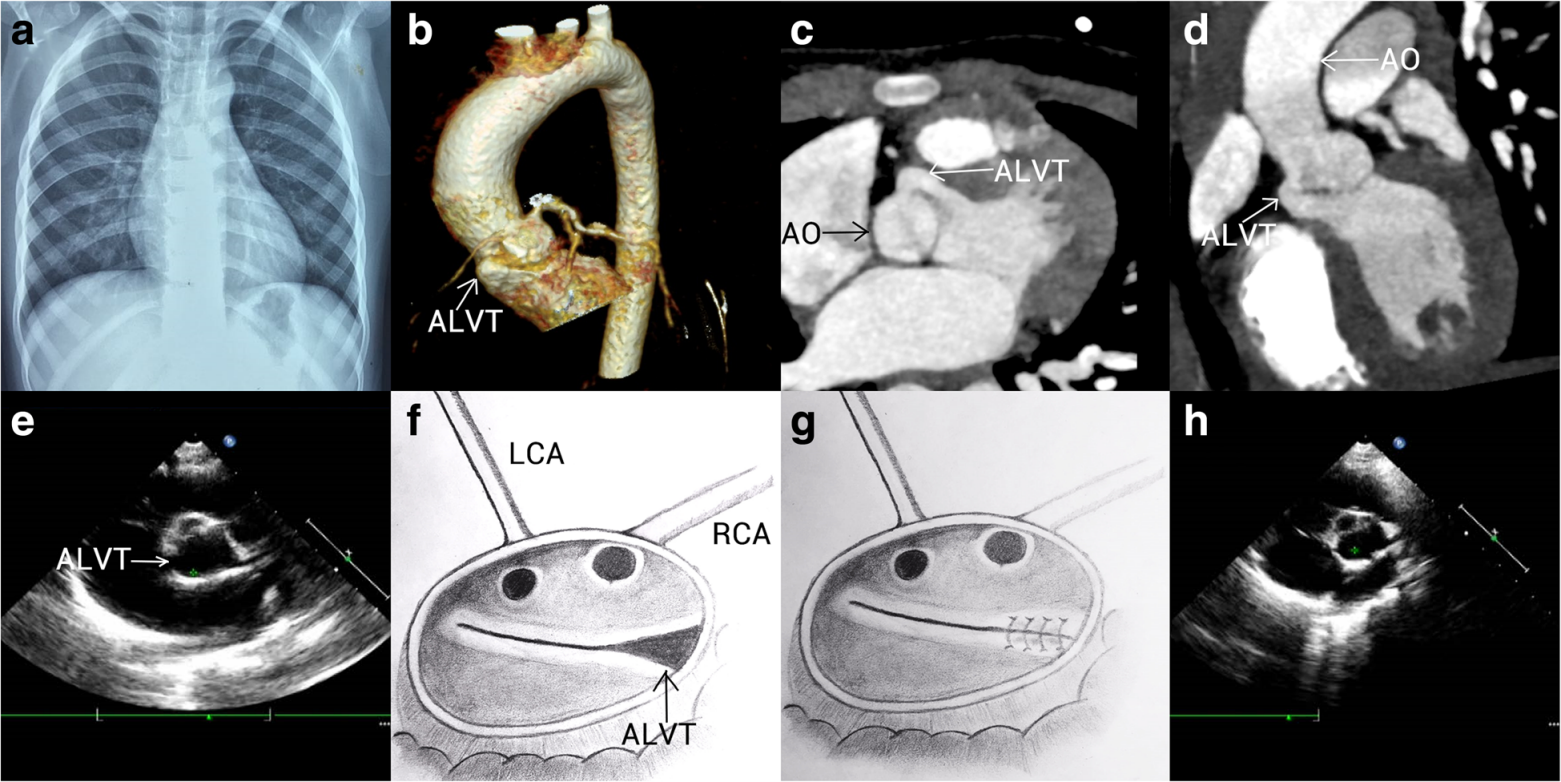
a The chest X-ray showed no significant aberrance. b-e The preoperative cardiac CTA, 3D reconstruction of cardiac CTA and echocardiography revealed an abnormal tunnel between the right posterior sinus and left ventricle. f-g Near anterolateral commissure the gap between the roots of two leaflets with a width of 0.6 cm formed into a special ostium with the inner wall of aorta when the leaflets were closed, which was sutured using 5–0 running polypropylene. h The postoperative echocardiography showed that the ALVT was turned off
